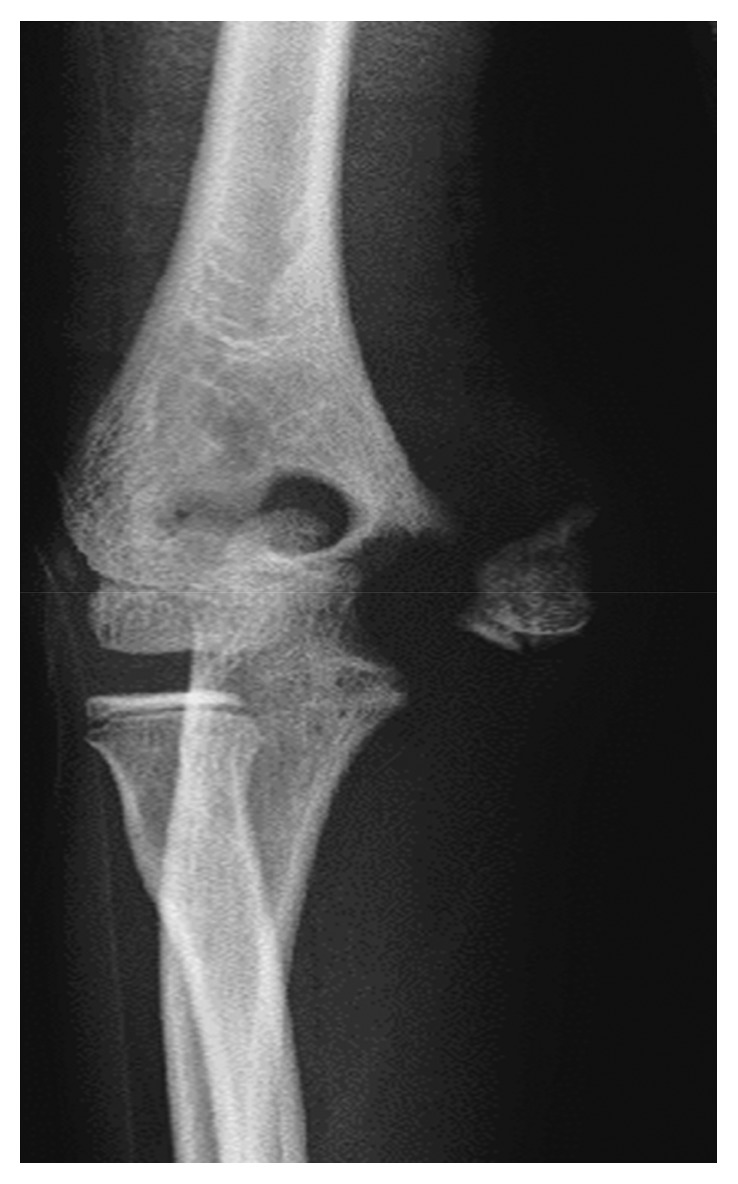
Anteroposterior radiographs of the right elbow at the initial visit. A medial condyle fracture (Kilfoyle type III) was seen.
